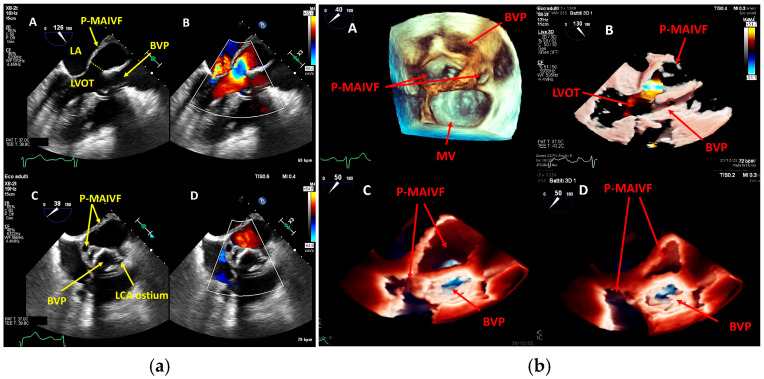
(a,b): On the left (a), a pseudoaneurysm of the Mitral-Aortic Intervalvular Fibrosa (P-MAIVF) is visible. The cavity demonstrates systolic expansion (A, C) and color Doppler flow (B, D) directed inward during systole. On the right (b), the 3D echo view is displayed (A: surgical view, B, C, D: “TrueVue Glass” view helps delineate the anatomical borders of the cavity). LCA = Left Coronary Artery. BVP = Biological Valve Prosthesis; LVOT = Left Ventricular Outflow Tract. LA = Left Atrium. This is an original, de-identified clinical image from our institution. Patient consent for publication was obtained.
